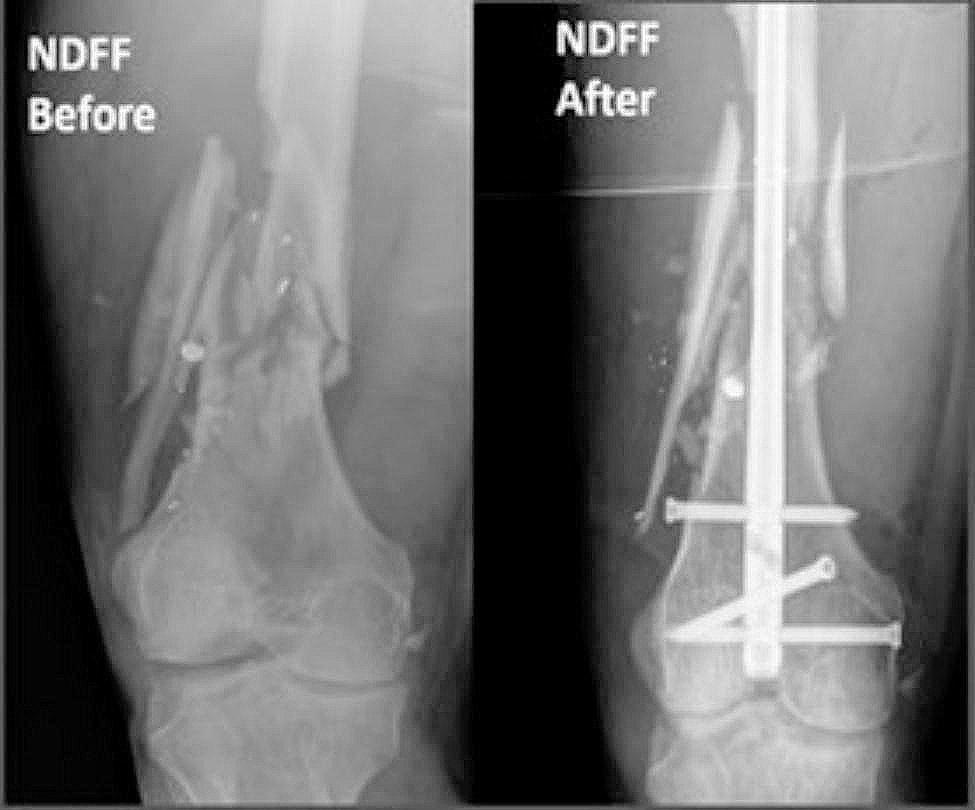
Preoperative (left) and post-IMN (right) NDFF radiographs
